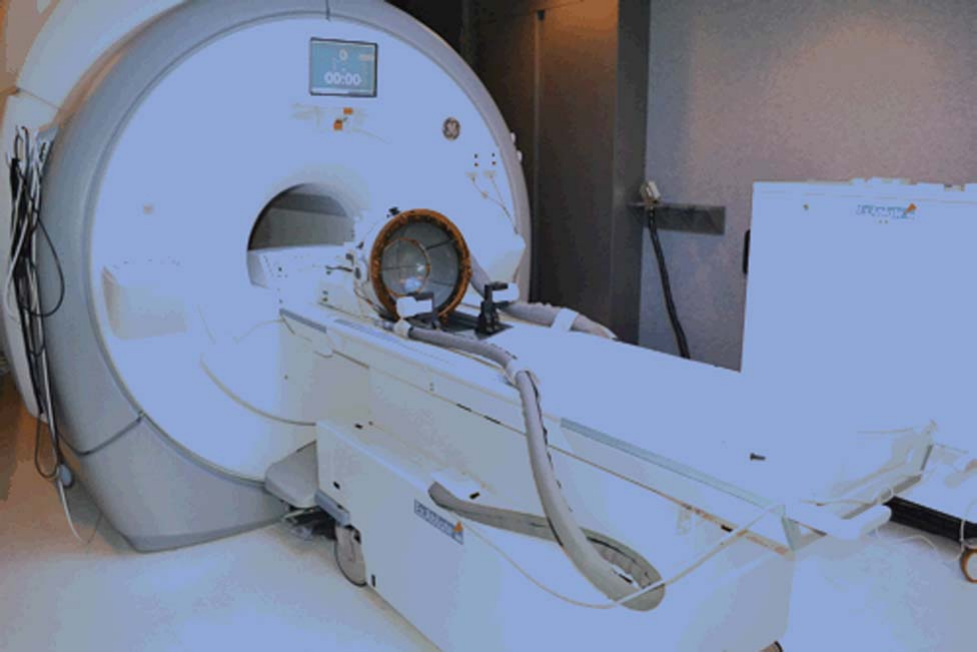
Commercially available magnetic resonance-guided (MR-guided) focused ultrasound.^10^
